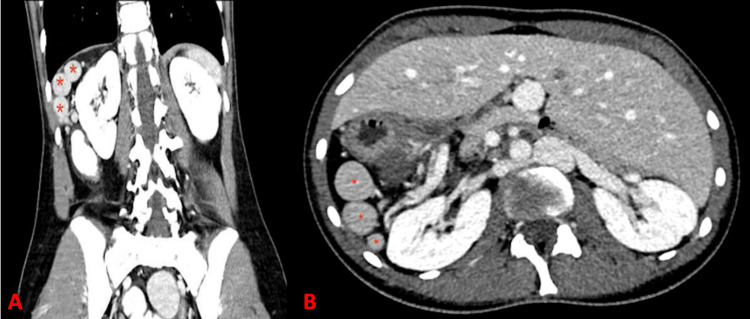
Abdominal CT scan at the time of admission shows multiple accessory spleens (red stars) in the coronal (A) and axial (B) views.
